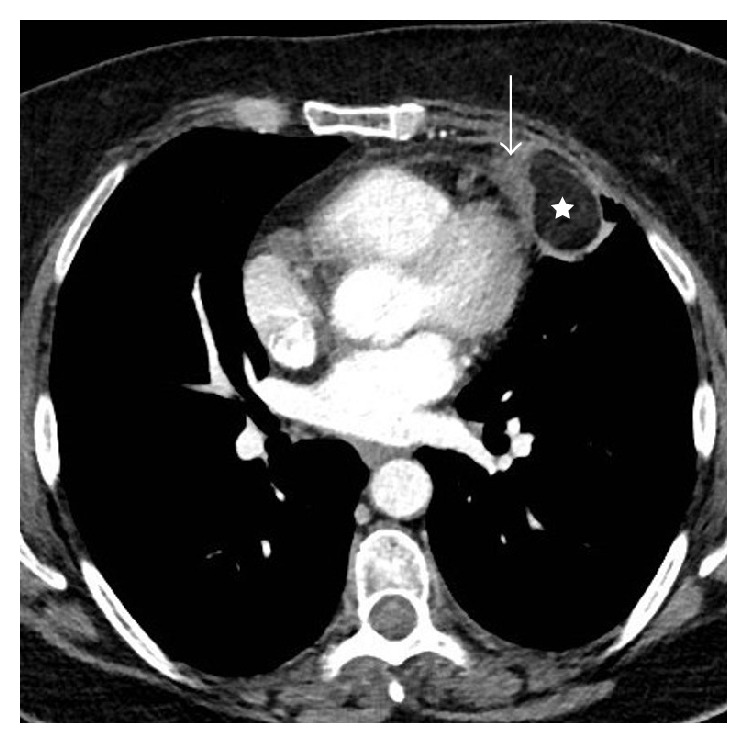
Contrast-enhanced CT showing that the central fatty attenuation of the mass is not enhanced (star); the capsule shows a 50 HU increase in density (arrow).
